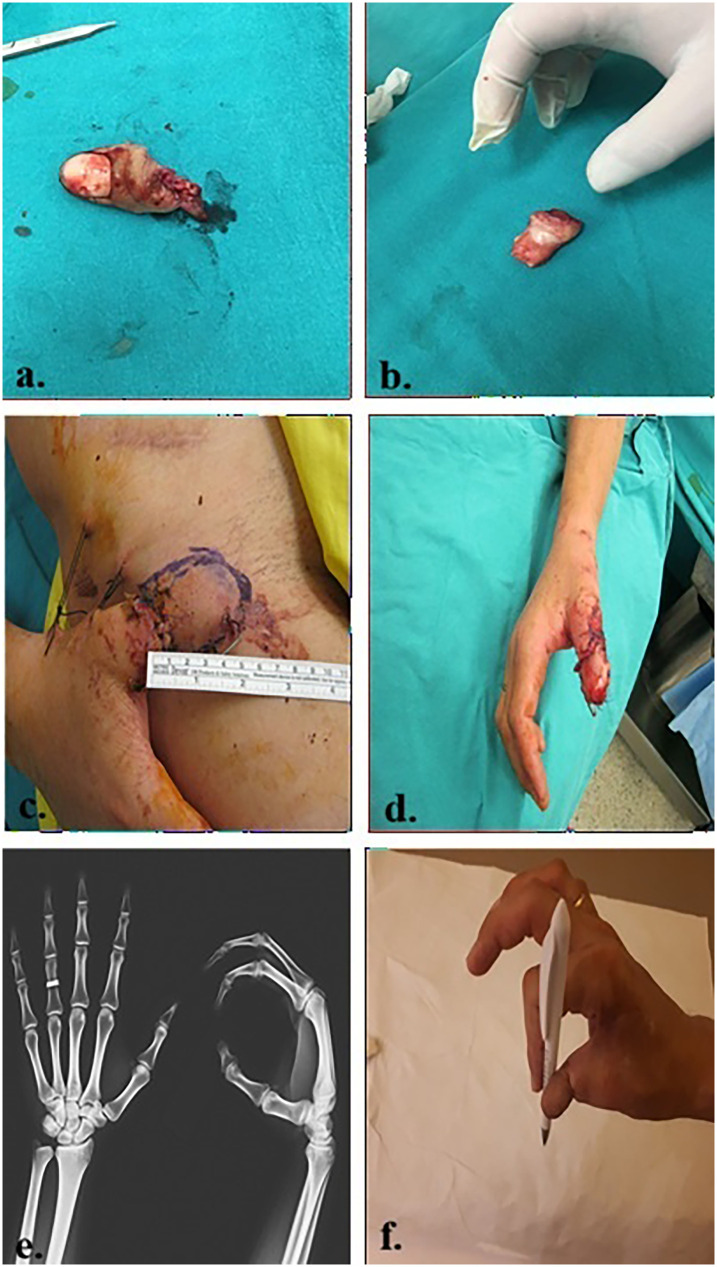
Clinical sequence demonstrating staged heterotopic groin-pocket salvage following intraoperative thumb replantation failure in a 42-year-old male patient with traumatic right-thumb amputation. (a) Amputated thumb following failed replantation attempt. (b) Debridement of the distal phalanx segment with preservation of the flexor pollicis longus (FPL) and extensor pollicis longus (EPL) tendon insertion sites. (c) Reopening of the groin pocket on postoperative day 10 for SEPRAFILM® application around the peri-interphalangeal region and temporary skeletal stabilization using Kirschner-wire fixation under ultrasound guidance. (d) Clinical appearance during staged reconstruction prior to pedicle division. (e) One-year postoperative radiograph showing mild joint-space narrowing without gross articular collapse or advanced degenerative change. (f) Demonstration of functional precision pinch at 12 months, showing the patient holding a pen with mild compensatory hyperextension.
